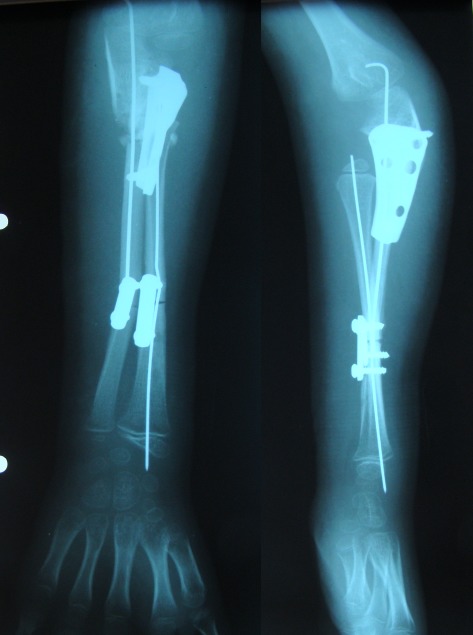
The final configuration of the forearm (postoperative radiograph). The fibular graft is fixed by using a plate with two screws and the two ulnar osteotomies are stabilized by the prosthesis and a plate with screws, respectively. Both bones are further stabilized by using a central pin or thin elastic rod
